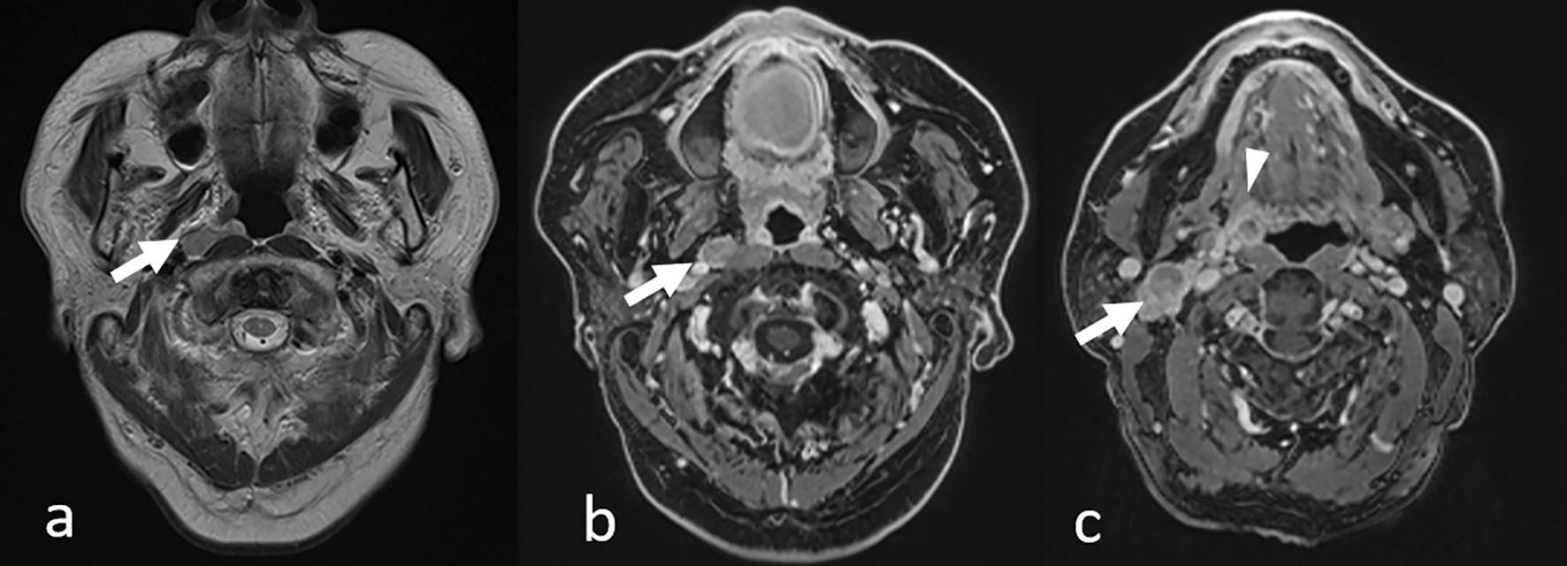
Incidentally detected mesopharyngeal carcinoma (p16 negative) with lymph node metastases in a patient with lung adenocarcinoma. T2-weighted image a shows right retropharyngeal lymph node metastasis as a hyperintense nodule in the retropharyngeal area (arrow). Contrast-enhanced 3D T1-weighted images with fat suppression of axial reconstruction (b and c) show an enlarged right retropharyngeal lymph node (b, arrow), right superior internal jugular nodule (c, arrow), and right mesopharyngeal tumor (c, arrowhead)
